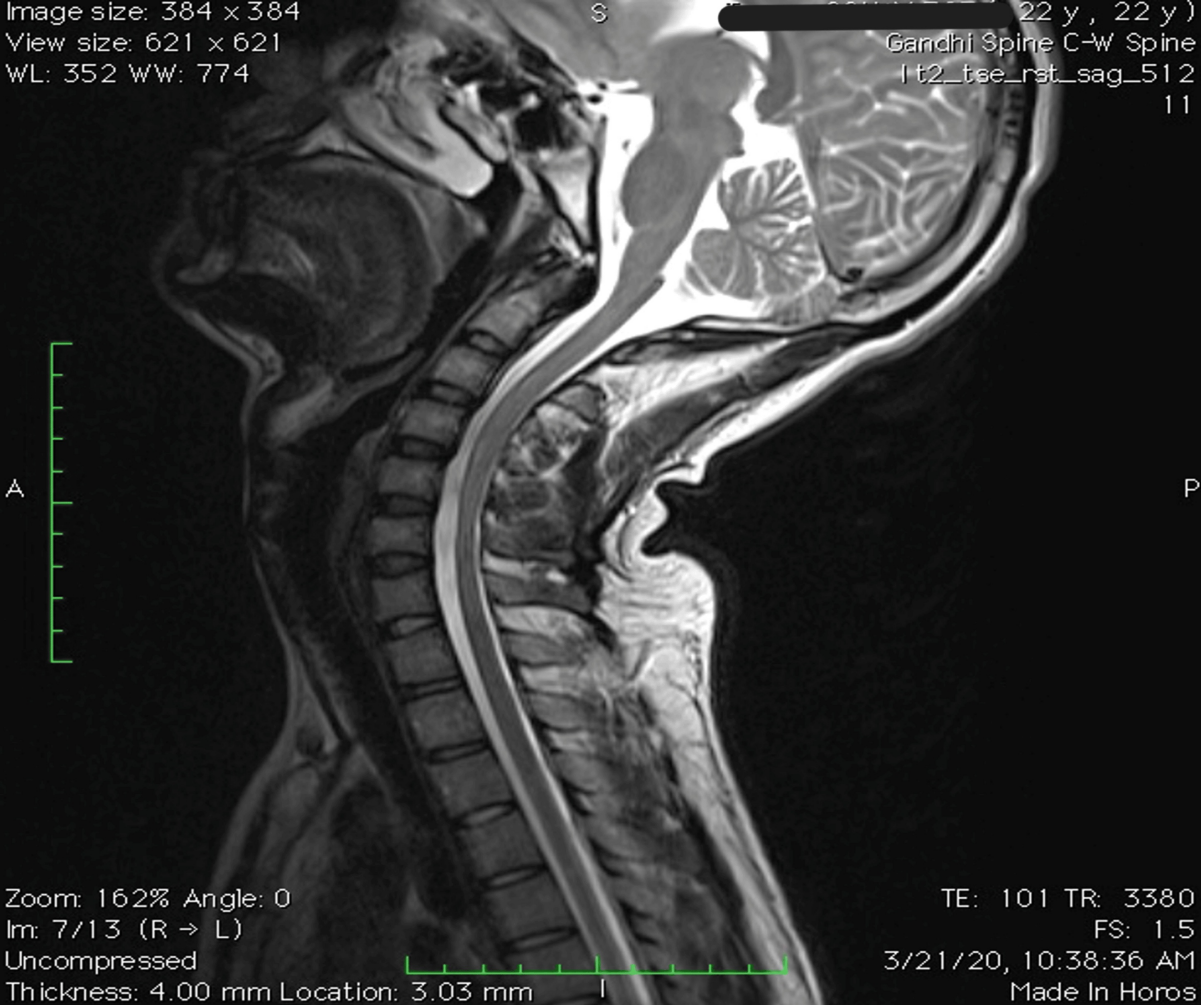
T2W sagittal MRI of the neck in extension; findings seen on flexion MRI are not visible here
